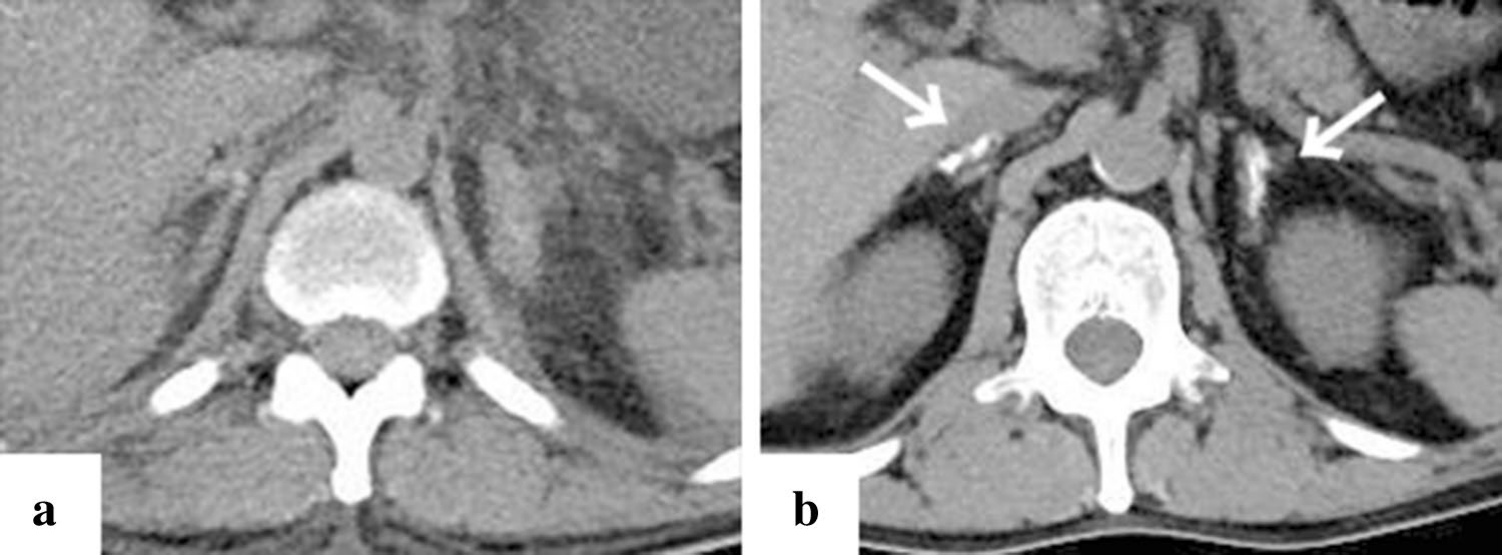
Representative case of adrenal calcification in a 50-year-old patient (case no. 2). Computed tomography (CT) imaging on day 41 since the onset of symptoms (a) and after one month of the admission (b). CT imaging on admission indicated adrenal enlargement (a), and CT one month later adrenal calcification (b, white arrow)
